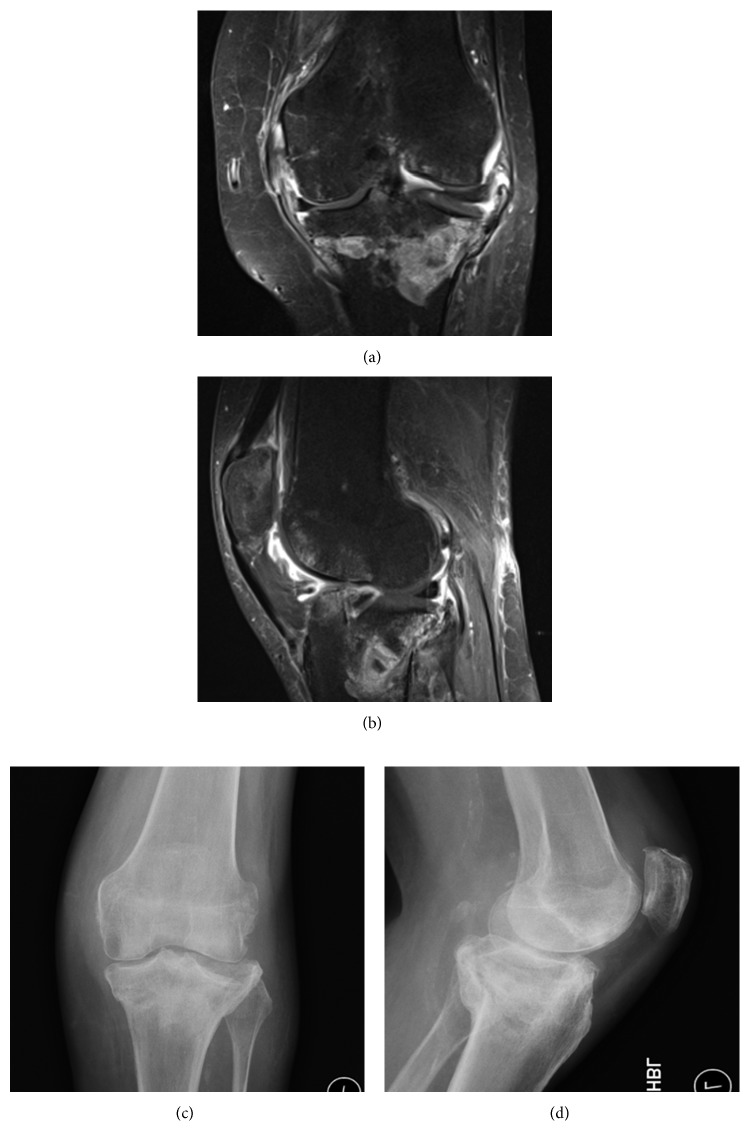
Post-Exogen therapy. (a) Coronal MRI T1 Fat Sat + Gad, (b) sagittal MRI coronal Fat Sat, (c) AP, and (d) lateral radiographs.
